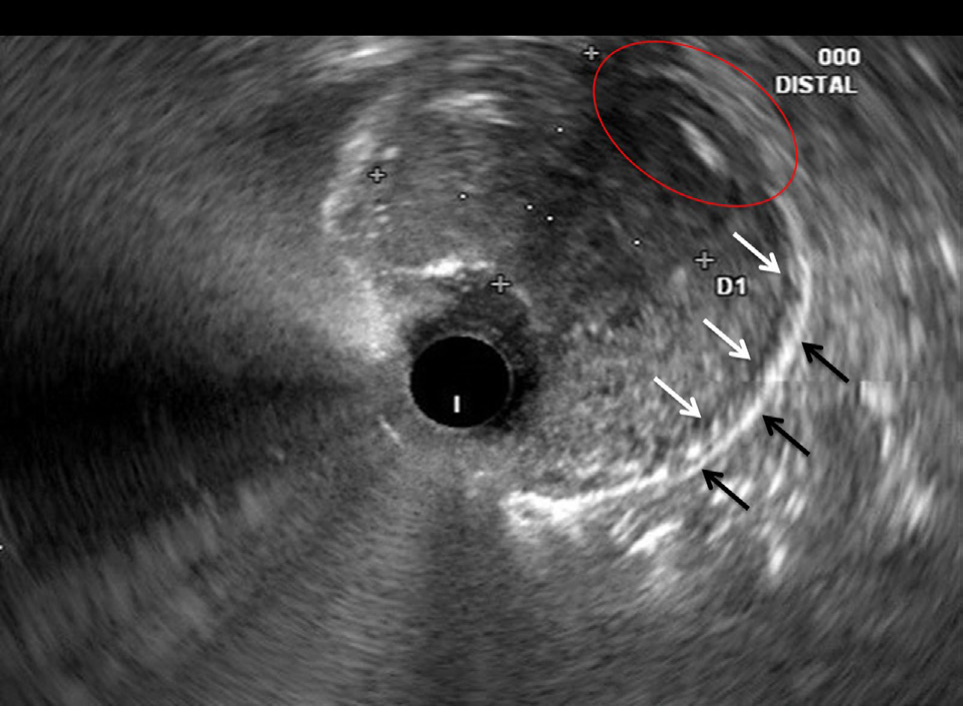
Correlative endoscopic ultrasound image revealing the muscularis propria (white arrows), serosa (black arrows), and an area in which the mass invades these layers (red oval). The mucosal and submucosal layers are obliterated by the mass and difficult to identify in this image.
